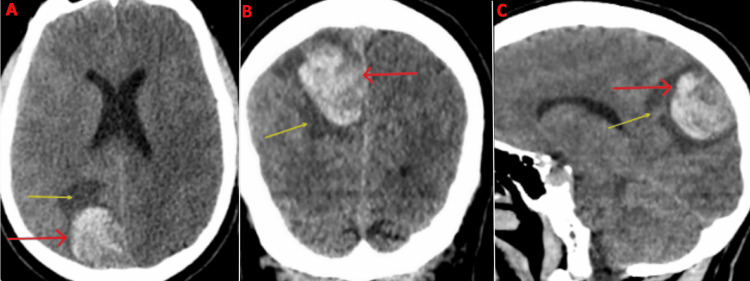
CT head on arrival to the emergency department with views of the axial (A), coronal (B), and sagittal (C) planes Red arrows: Hyperdense mass, Yellow arrows: Vasogenic edema
